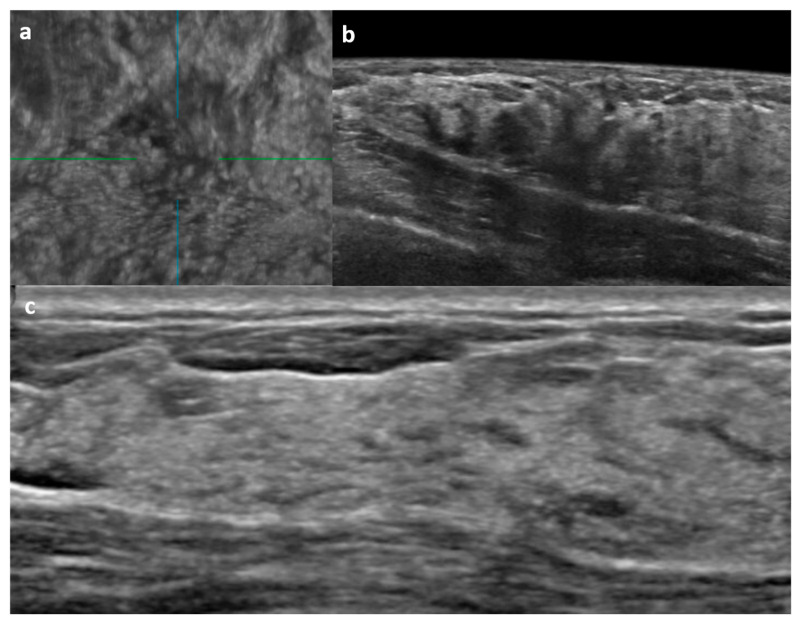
A 54-year-old woman diagnosed with a transient non-mass ABUS finding due to an inadequate compression artifact. (a,b) The axial and coronal planes of ABUS show a non-mass lesion with posterior shadowing, ≥50% FGT involvement, and no volume change in the upper outer quadrant of the right breast. (c) Second-look ultrasound performed subsequently in the same region does not clearly demonstrate a corresponding focal lesion.
